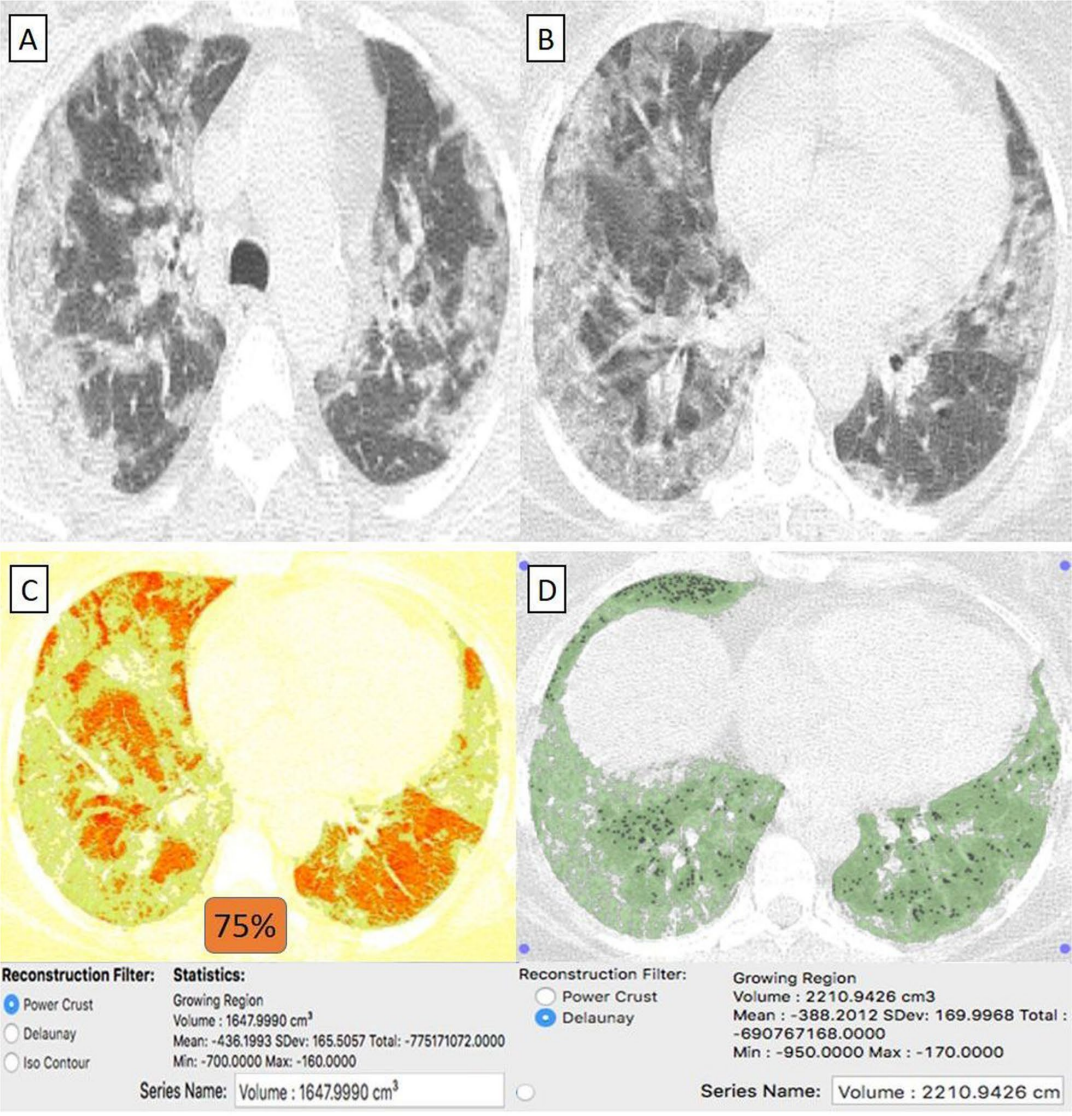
A 55-year-old female patient with history of systemic lupus erythematosus (SLE) and had received “Sinopharm” vaccine then got COVID-19 infection. A, B Axial chest CT cuts (lung window) showed bilateral mainly peripheral located wide spread ground-glass pneumonic patches. C CT-Volumetry of the pathological lung parts averages 1647 cc. D CT-Volumetry of total lung volume averages 2210 cc. So, 75% of the lung parenchyma was diseased (Score 3)
